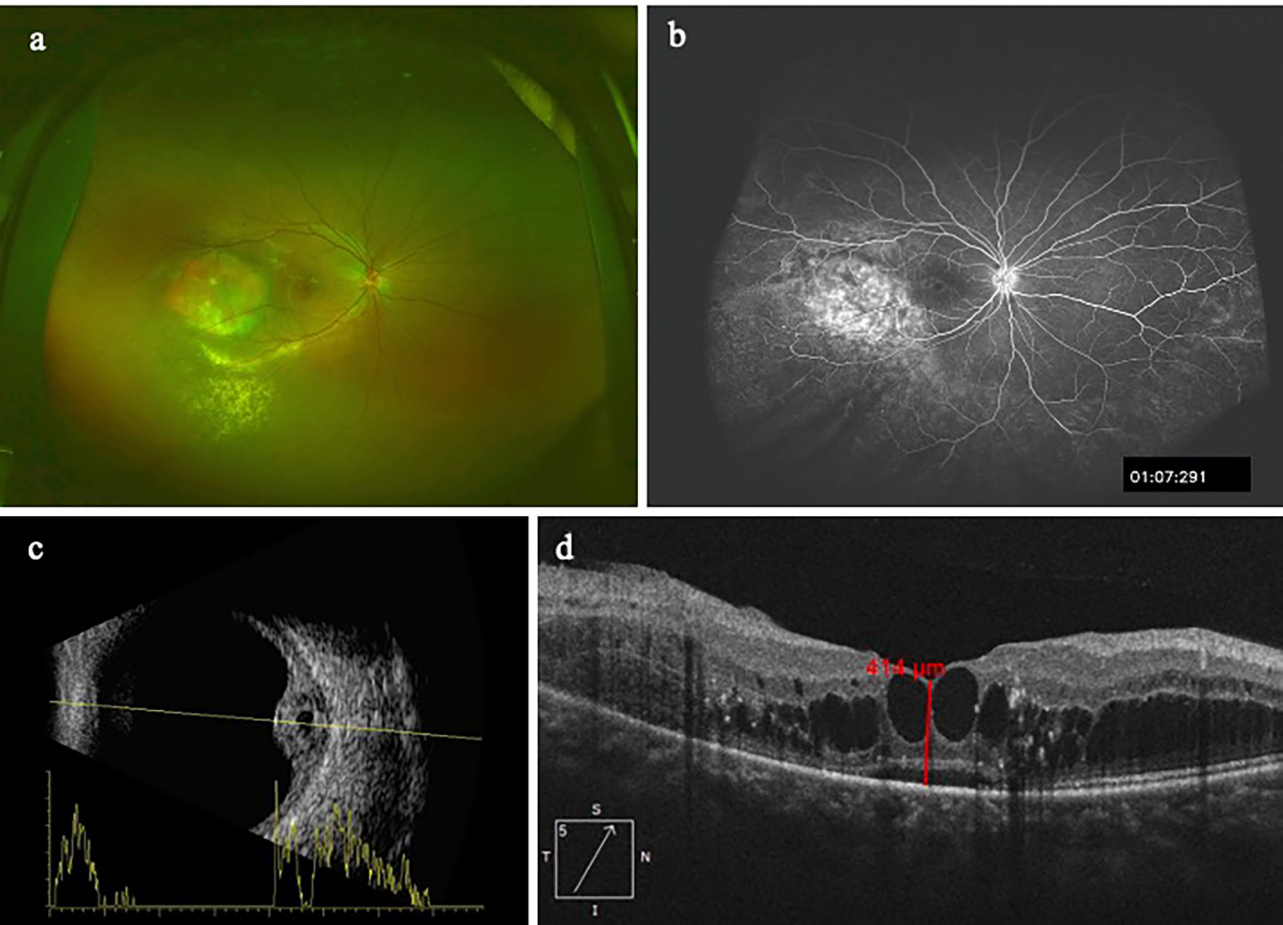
A patient with CCH. The concentration of IP-10 in aqueous humor is 76.4 pg/ml while the concentration of VEGF-A is 987.57pg/ml. (A) CCH temporal to the fovea; (B) The FFA clearly delineates the hemangioma with leakage; (C) Ultrasonography reveals a tumor thickness of 4.3mm; (D) OCT image shows intraretinal cystic edema and subretinal fluid.
